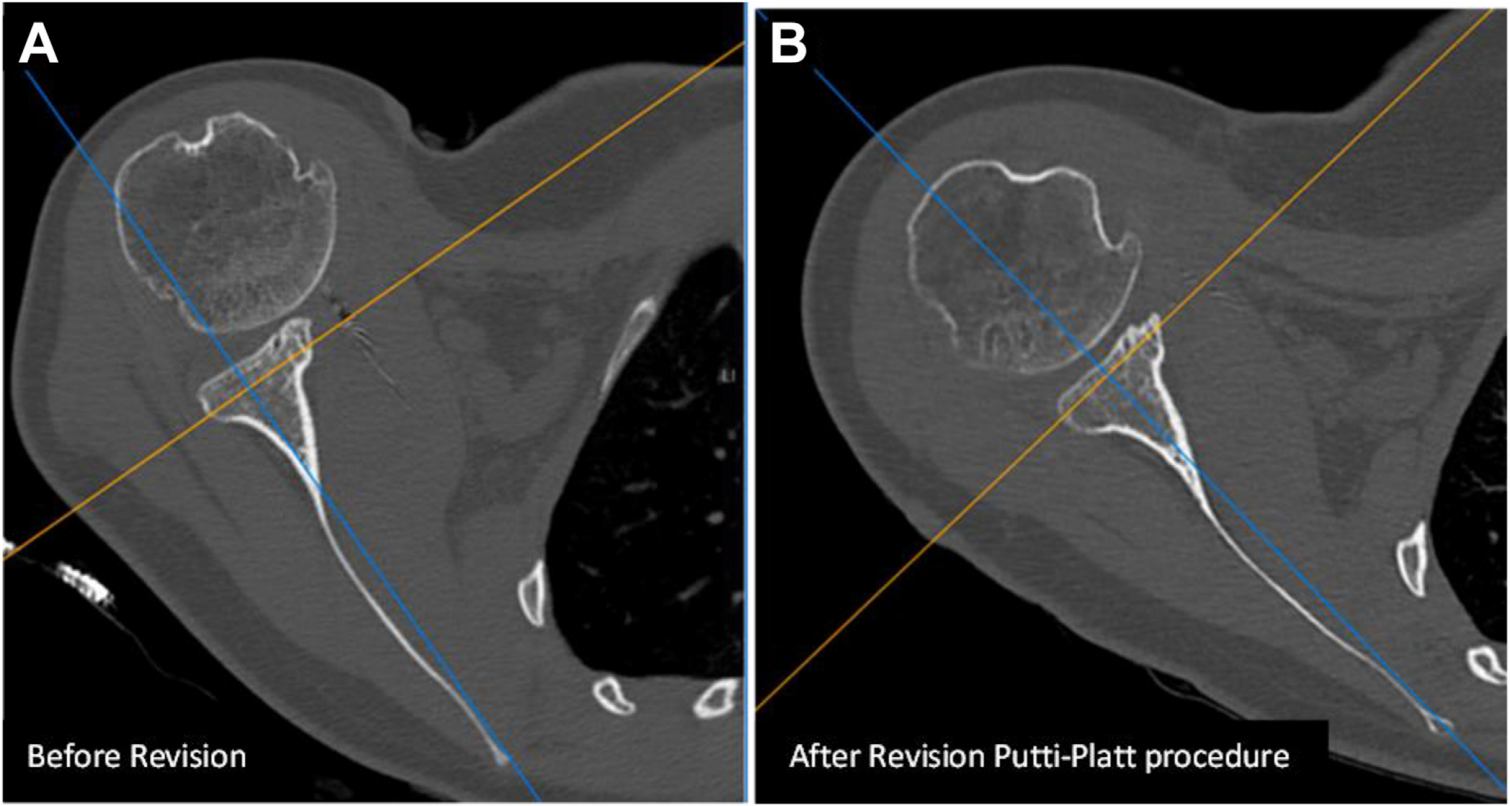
Comparison of preoperative and postoperative 2-dimensional Axial CT scan images. (A) Preoperatively, there is anterior subluxation of the humeral head despite good positioning and healing of coracoid bone block, absence of Hill-Sachs lesion and normal subscapularis muscle and (B) postoperatively, the humeral is recentered in front of the glenoid surface. Notice that the subscapularis muscle did not deteriorate, and osteoarthritis did not increase. *CT*, computed tomography.
